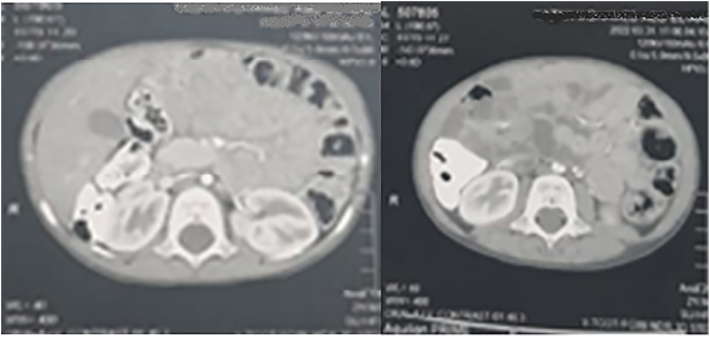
A cluster of small intestine loops was seen at the left anterior para-renal space on the postcontrast CT abdomen and pelvis axial plane.
